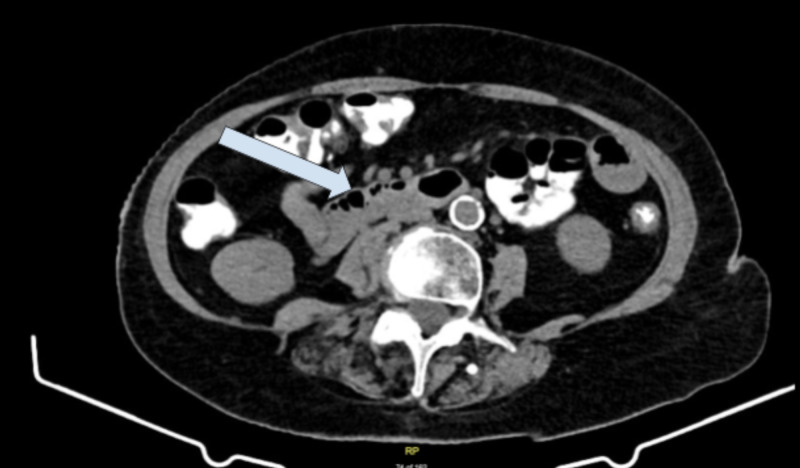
CT of the abdomen/pelvis with contrast No bowel wall thickening or obstruction. No mesenteric stranding or free fluid.
